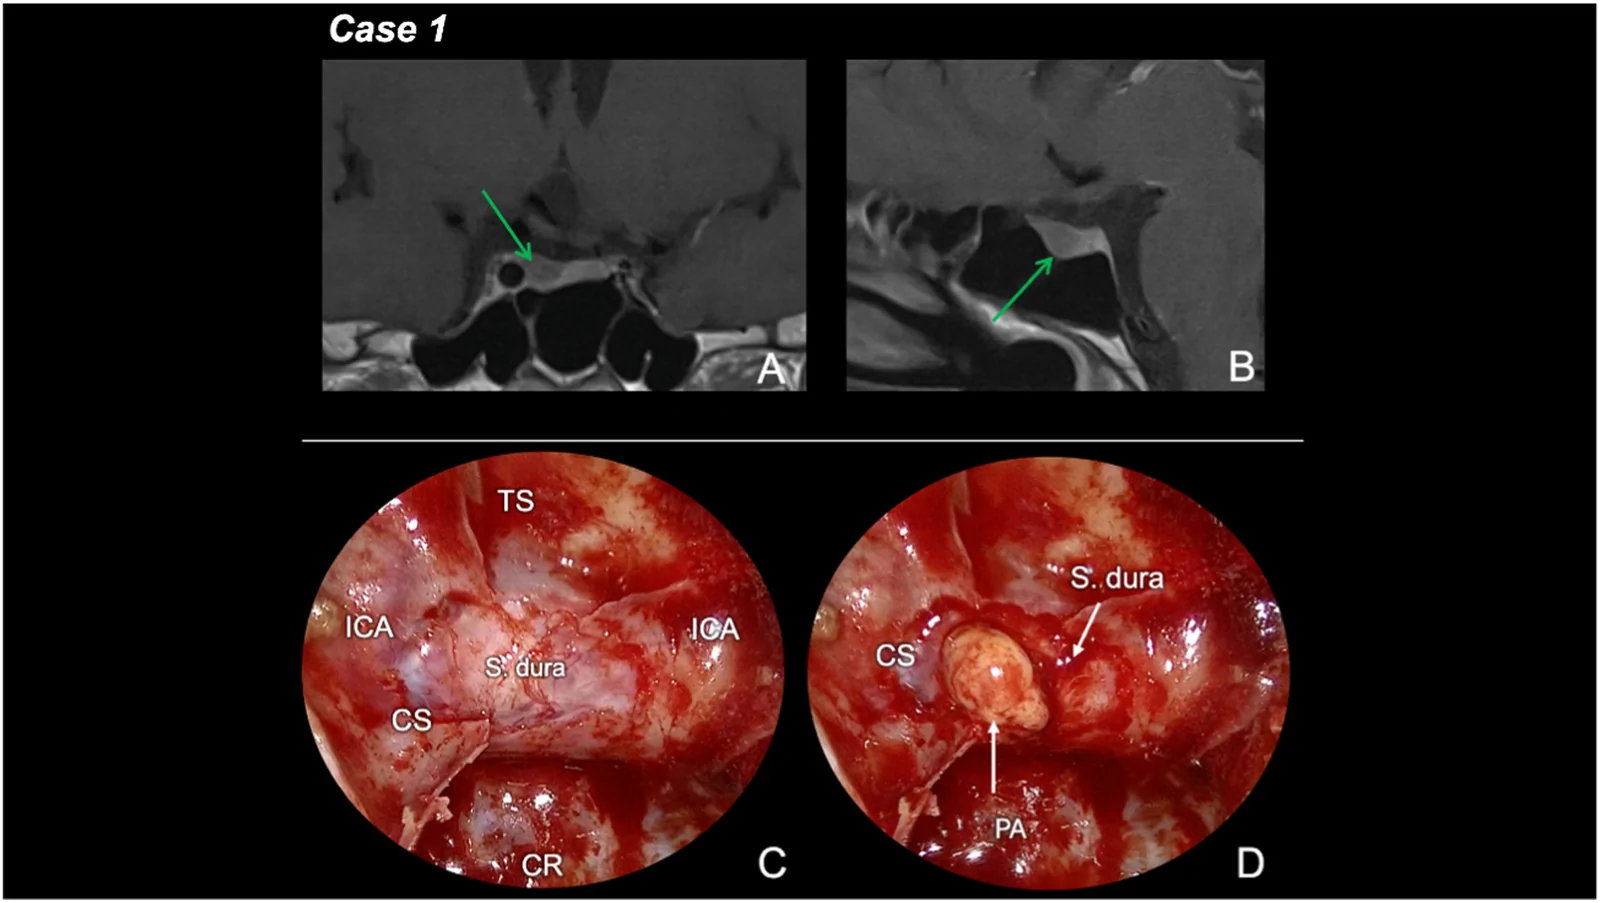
MRI-positive with a paracavernous microadenoma (Case 1) (A–B) Contrast-enhanced T1-weighted pituitary MRI showing an 8-mm right paracavernous ACTH-secreting microadenoma (green arrows). (C–D) Intraoperative endoscopic views demonstrating sellar opening, dural incision, and pituitary adenoma (PA) identification. *Abbreviations*: ICA: internal carotid artery; CS: cavernous sinus; S.dura: sellar dura; CR: clival recess; TS: tuberculum sellae.
